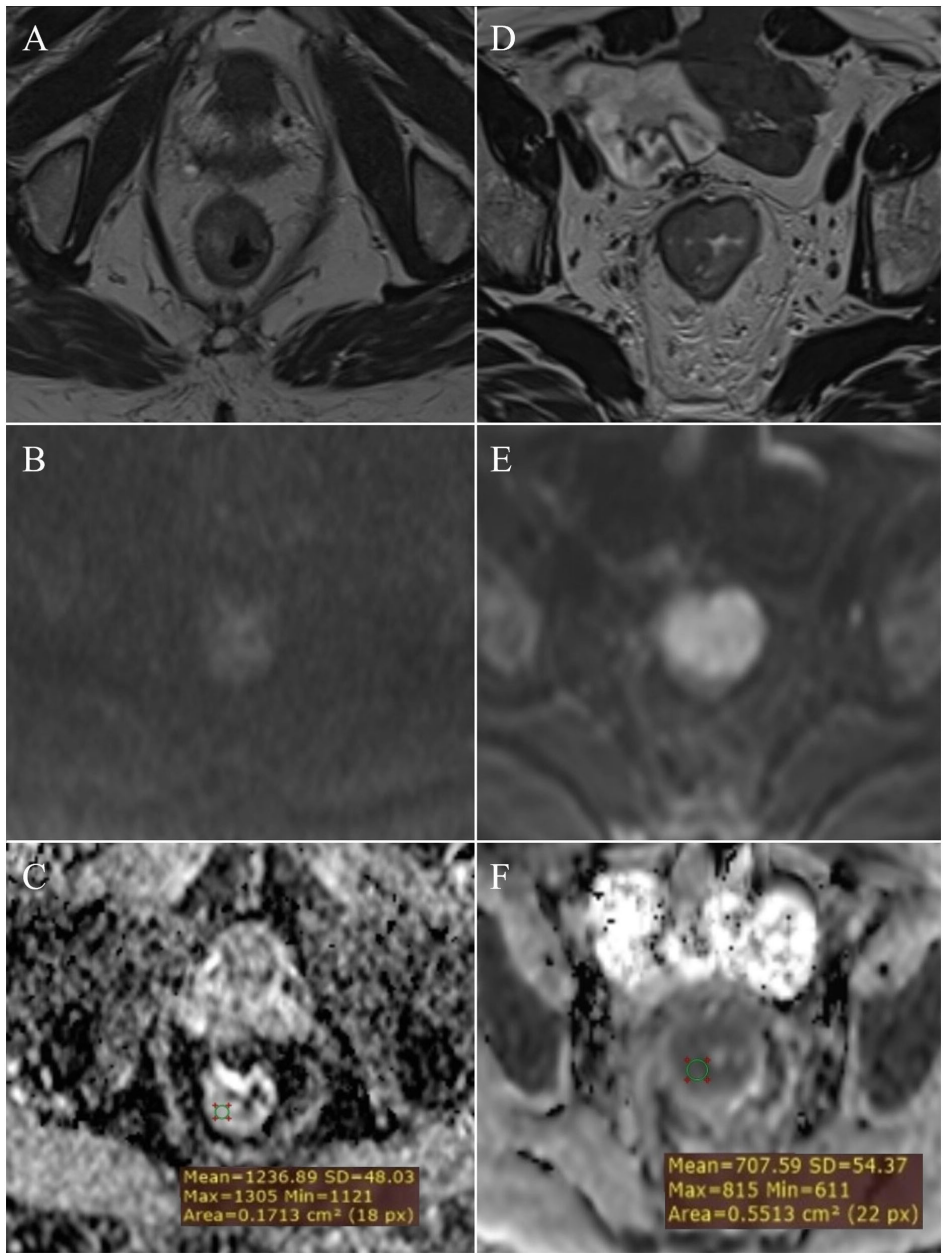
Measurement of ADC values: normal rectal wall – (A) (T2W), (B) (DWI), (C) (ADC map with ROI); T2 stage of rectal cancer - (D) (T2W), (E) (DWI), (F) (ADC map with ROI).
